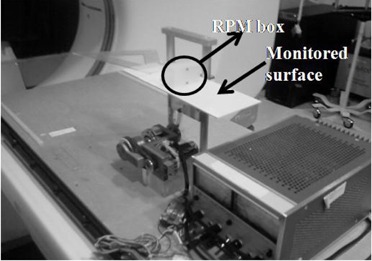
Experimental setup for the assessment of the calibration of CT scanner and optical systems for 4D image reconstruction.
